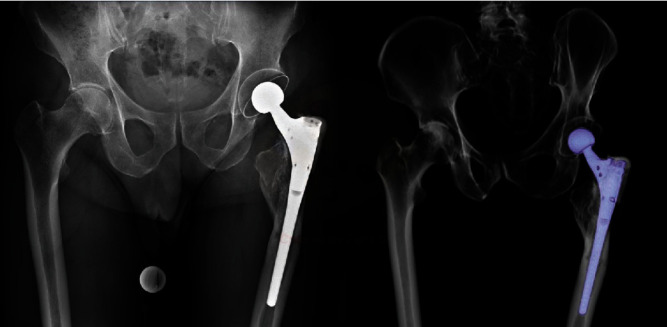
Postoperative AP pelvis X-ray and 3D CT scan showing a well-fixed cemented neotrochanter around the proximal part of the femoral component protecting the sharp edges of the stem.
